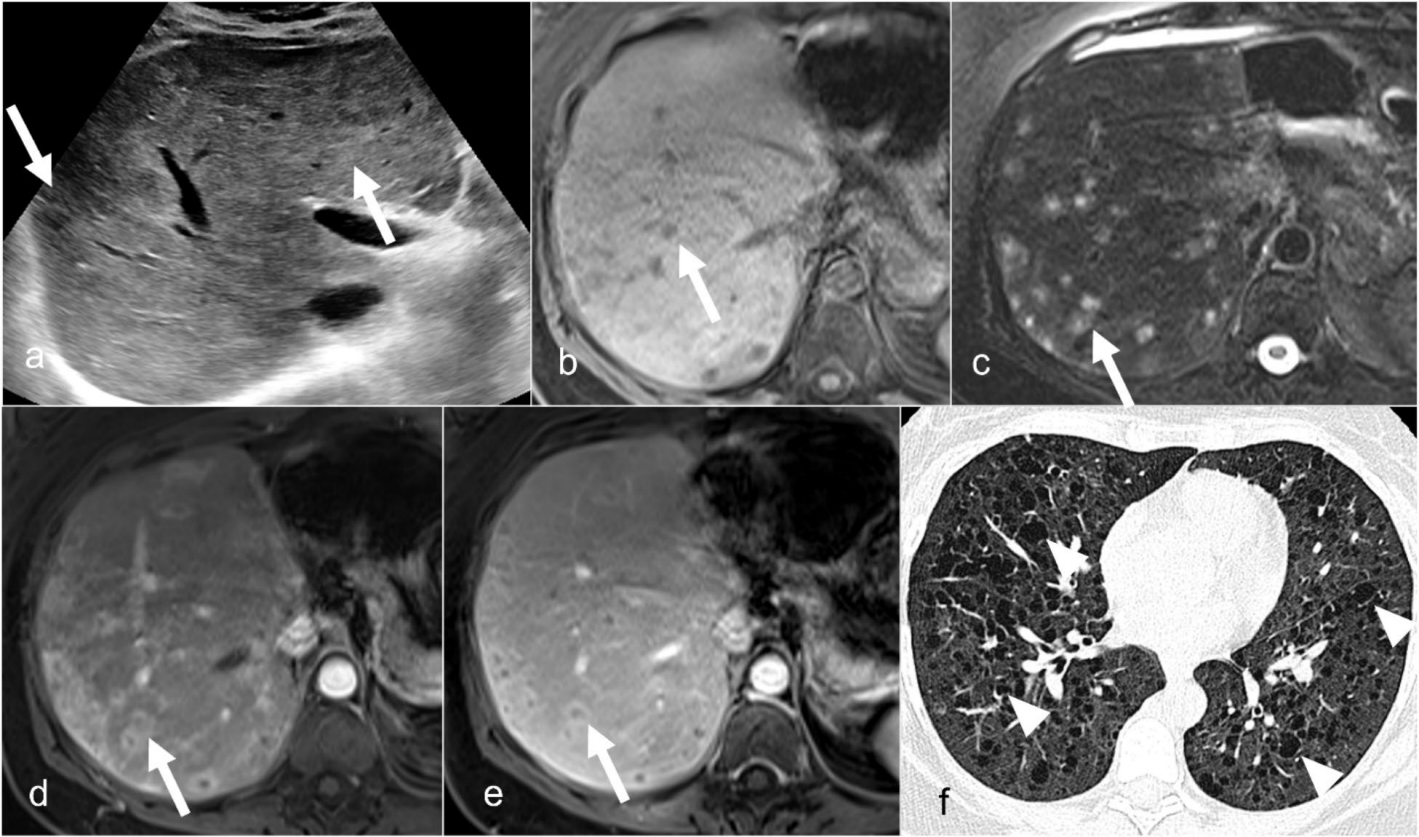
Langerhans cell histiocytosis in a 48-year-old female patient with known involvement of the temporal bone and lung. Grayscale ultrasound image (a) demonstrates heterogeneous liver parenchyma with multiple hypoechoic lesions (arrows). Axial MRI T1-weighted (b), T2-weighted fat-saturated (c), and postcontrast T1-weighted arterial and venous phase (d, e) images show T1 hypointense and T2-weighted hyperintense subcentimeter lesion with peripheral rim enhancement (arrows). Axial CT of the lung shows multiple small thin-walled cysts (arrowheads)
